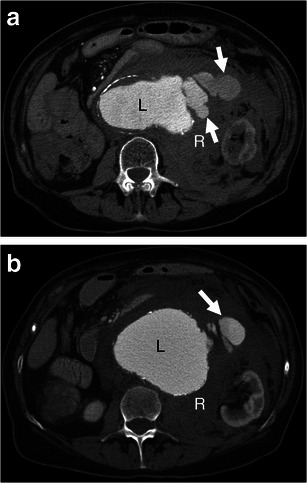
Contrast extravasation. a-b Axial enhanced CT of a 75-year-old woman demonstrates active contrast extravasation (*white arrows*) from the aneurysm lumen (*L*) into the retroperitoneal space with massive retroperitoneal haematoma (*R*)
